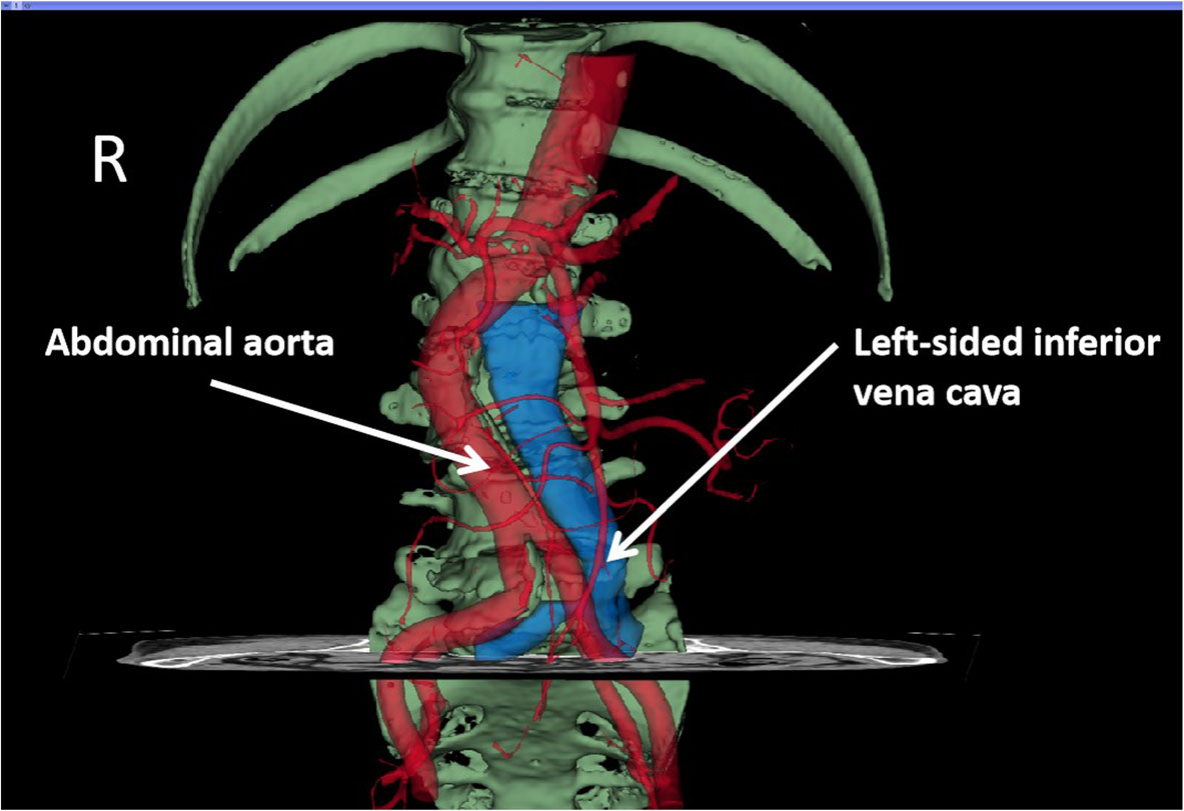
A reconstruction computed tomography angiography of retroperitoneal vessels and lumbar vertebrae indicated that the inferior vena cava was located on the left side
